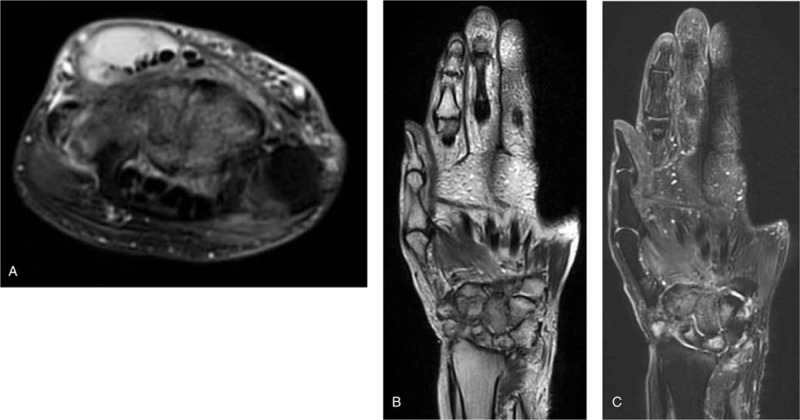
Preoperative MRI of the right wrist. (A) An MRI T2 weighted contrast transverse image reveals diffuse subcutaneous edema in the dorsum of the wrist and fluid collection in the extensor tendon sheath. Coronal image demonstrates the bone marrow edema with low signal intensity in the T1 WI (B) and high signal intensity in the T2 WI (C) in the bone marrow of the carpal bones. MRI = magnetic resonance imaging, WI = weighted image.
